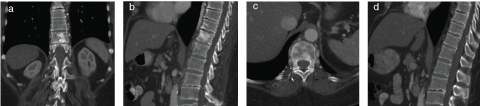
(a–d) A 66-year-old female with a history of lung and breast cancer presents with new onset abdominal pain. Contrast-enhanced CT scan of the abdomen and pelvis (a–c) reveals abnormal high attenuation within the lower thoracic vertebrae. Comparison was made with a prior study performed in 2011 (d), which showed no bone abnormality at that time. This was interpreted as new osseous metastatic disease.
